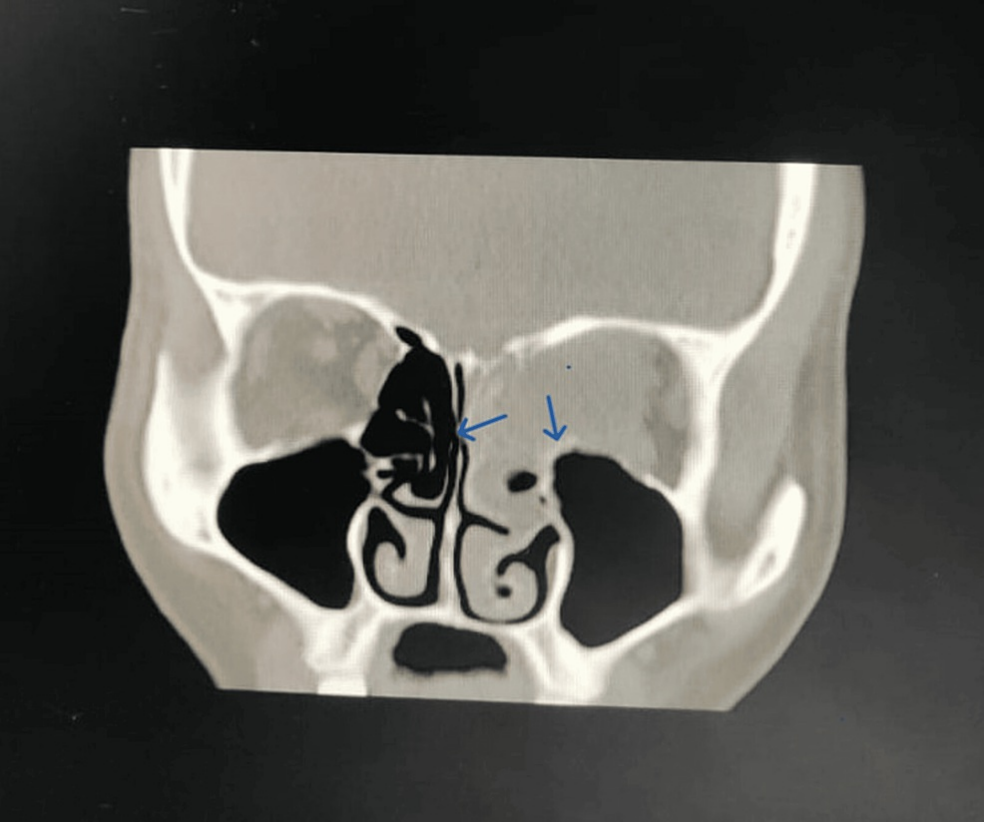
CT of the brain showing soft tissue attenuation mixed with air in the left ethmoid air cells, as well as bony destruction of the left lamina papyracea with a 22 x 10 mm extraconal soft tissue collection in the left orbit
